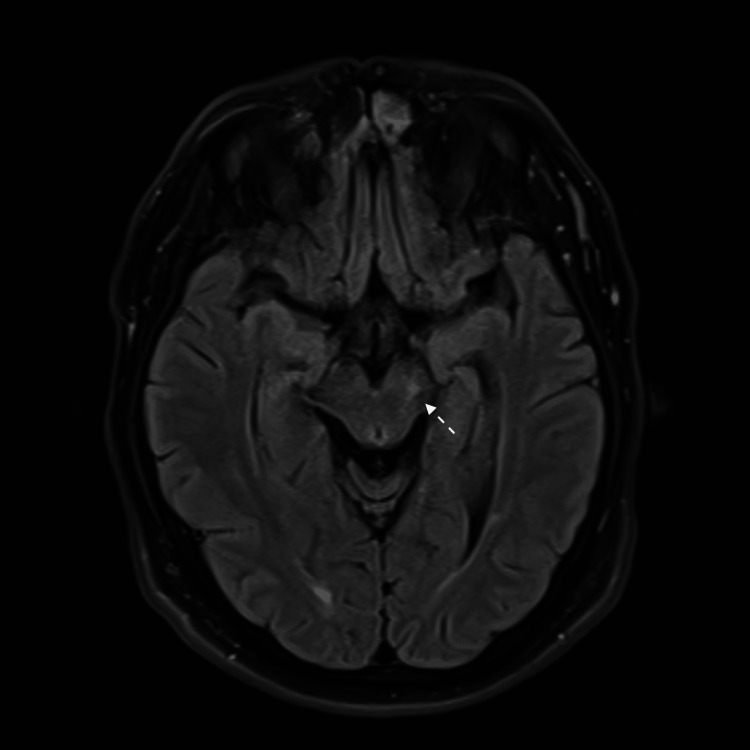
MRI brain FLAIR view shows left midbrain cerebral peduncle infarction.
